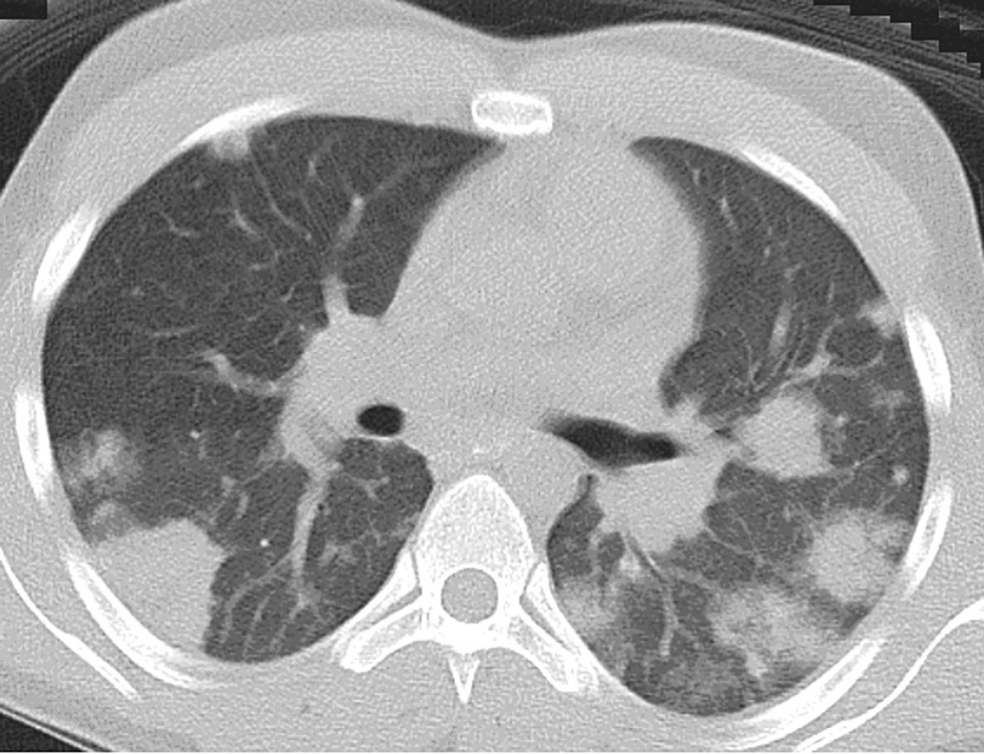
CT axial of the chest with IV contrast Select axial chest CT of the same patient delineates peripheral nodular and wedge-shaped opacities throughout both lungs corresponding to septic emboli CT: computed tomography; IV: intravenous
